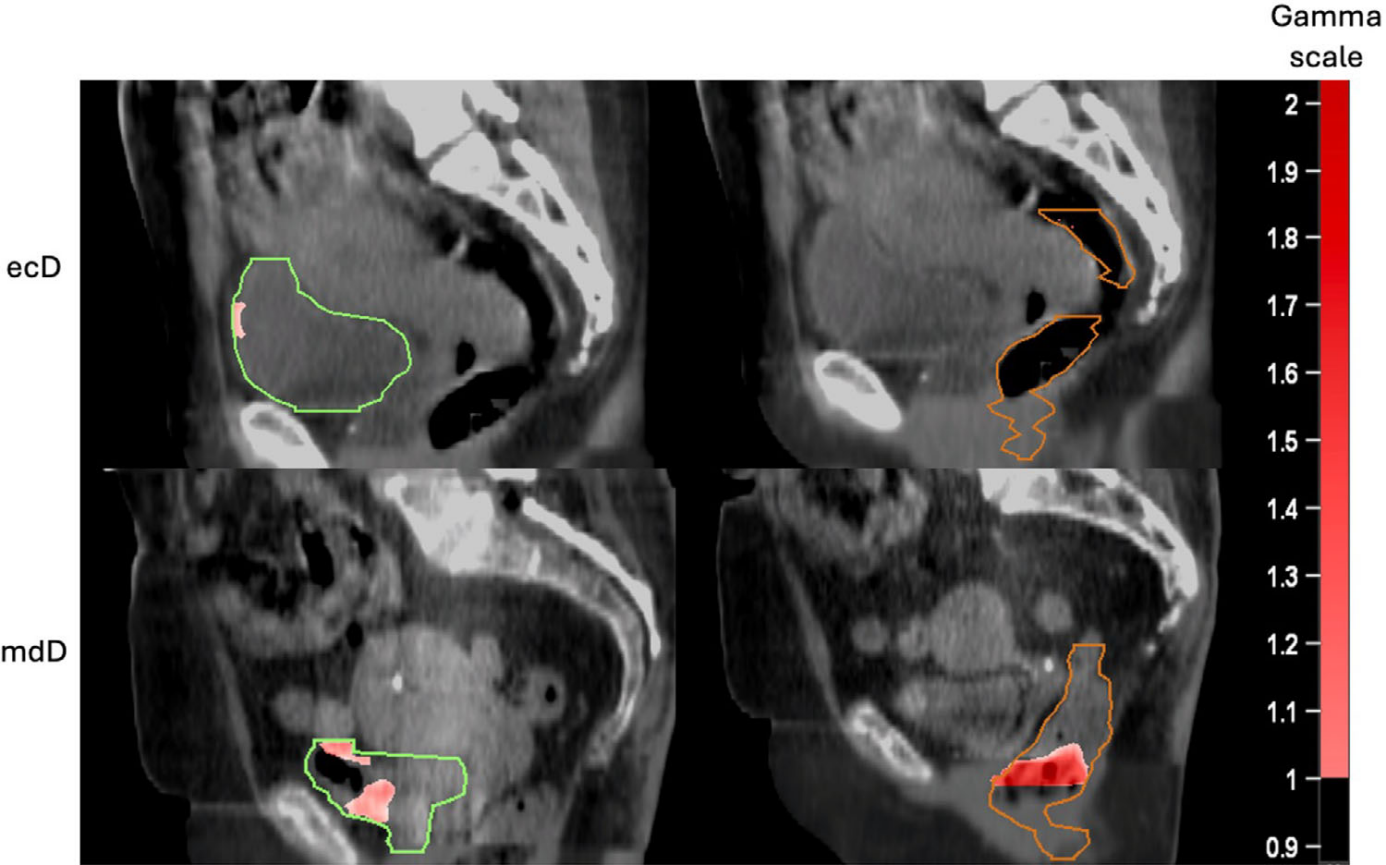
The passing gamma analysis results between pD and ecD are shown on the top row for patient 1 and the failing gamma analysis results between pD and mdD are shown on the bottom row for patient 20. The reference structure set is mCBCT1. The bladder is shown in green and rectum in brown.
